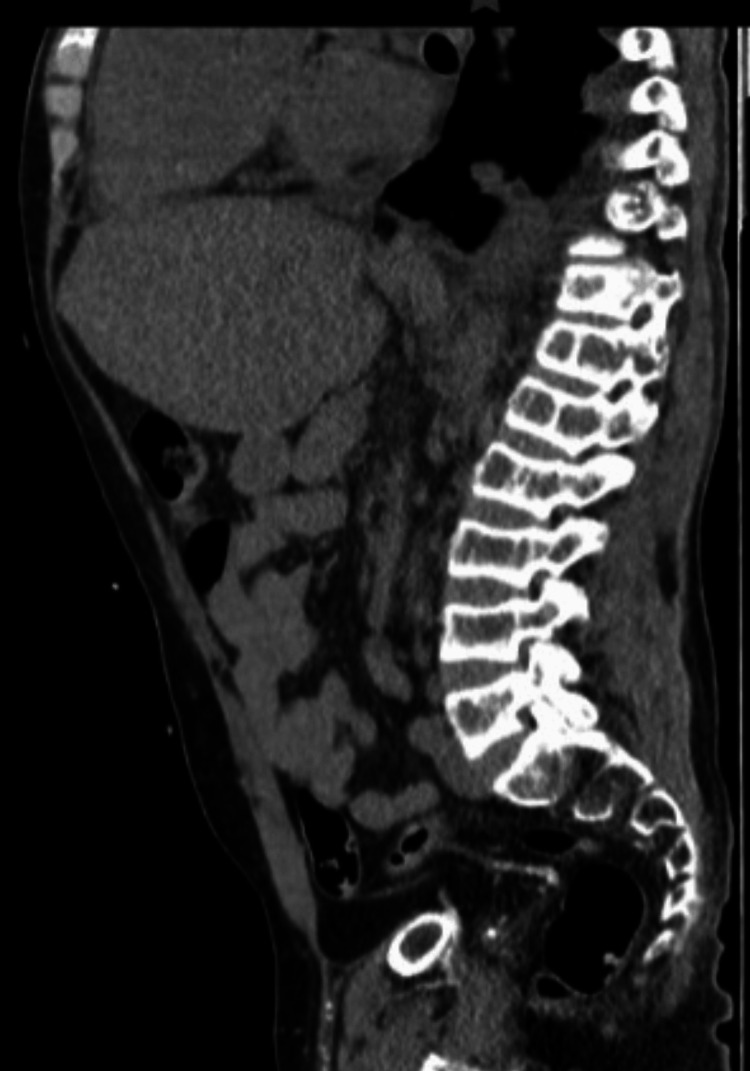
Sagittal non-contrast axial CT Sagittal section showing osteolysis, osteoporosis, and sclerosis in the lumbar vertebrae CT: computed tomography
